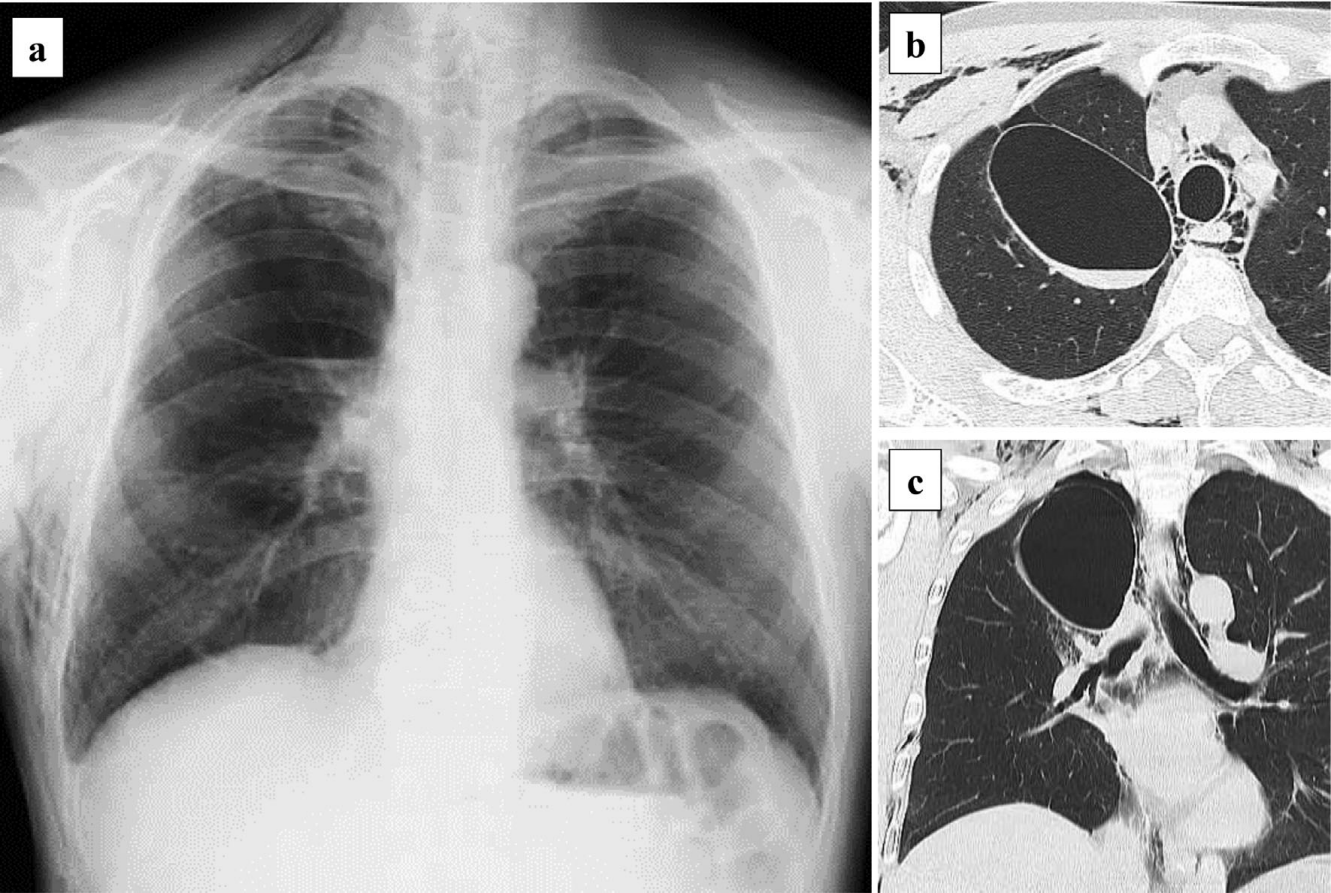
Imaging of the pulmonary cyst that developed rapidly. (a) Chest radiography depicting extensive subcutaneous emphysema, suggesting a pulmonary cyst in the upper right lung field. (b, c) Computed tomography depicting subcutaneous emphysema and mediastinal emphysema and an 8 cm fluid‐filled pulmonary cyst in the right lower lobe.
